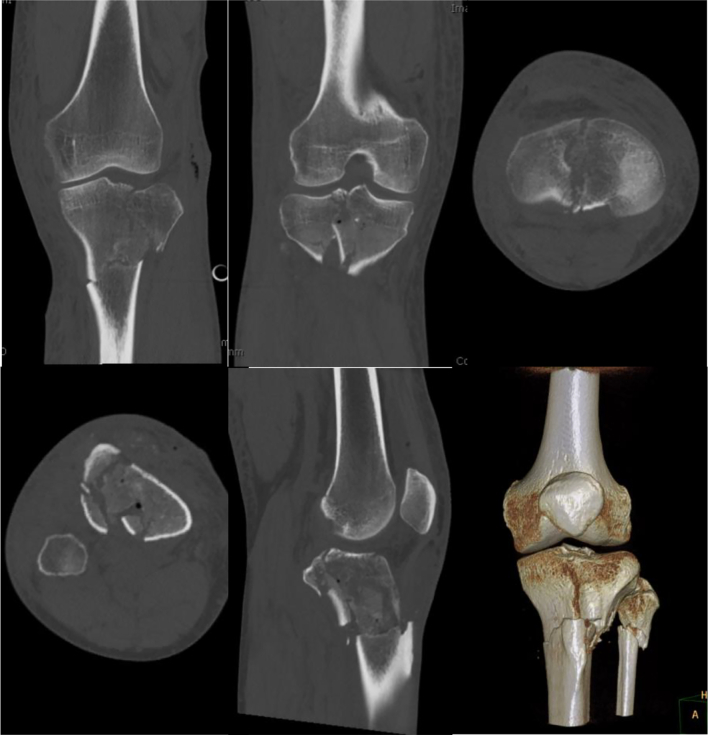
Coronal, axial, sagittal, and 3D reconstruction computed tomography images of Case 13, a 41C3/42A3 proximal tibial fracture component.
